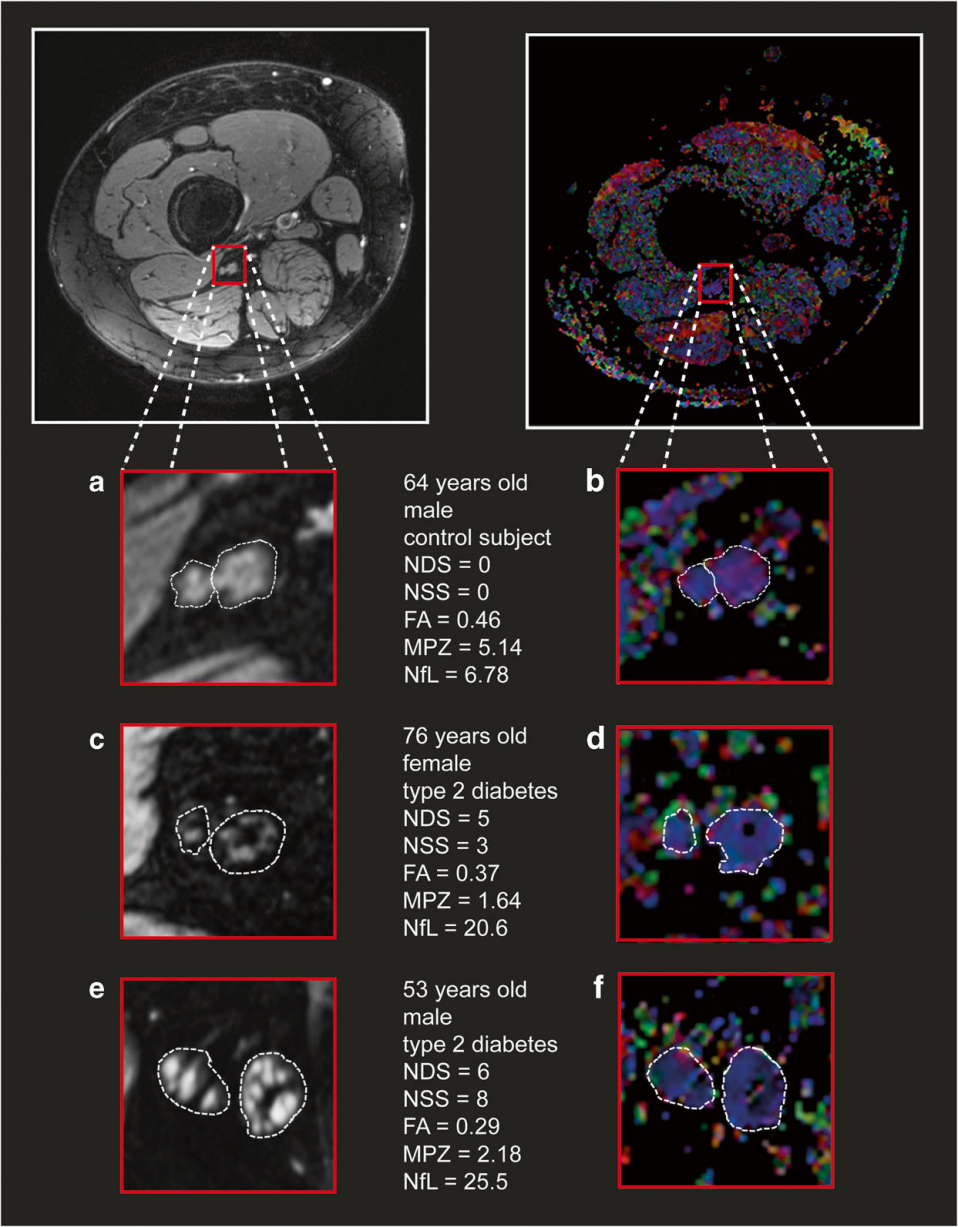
FA displays the integrity of nerve fibre mass in human sciatic nerve. (a) T2-weighted, fat-suppressed image of tibial and peroneal compartments of the sciatic nerve in a healthy control participant. (b) Colour-coded map of the FA of tibial and peroneal compartments of the sciatic nerve of the same participant as in (a). (c) T2-weighted, fat-suppressed image of the sciatic nerve showing fat-equivalent, hypointense fascicular lesions of the tibial and peroneal compartments in a participant with DPN. (d) Colour-coded FA map of the tibial and peroneal compartments of the sciatic nerve of the same participant as in (c). (e) T2-weighted, fat-suppressed image of the sciatic nerve showing both hyperintense and hypointense fascicular lesions of the tibial and peroneal compartments in a male participant with severe DPN. (f) Colour-coded FA map of tibial and peroneal compartments of the sciatic nerve of the same participant as in (e)
